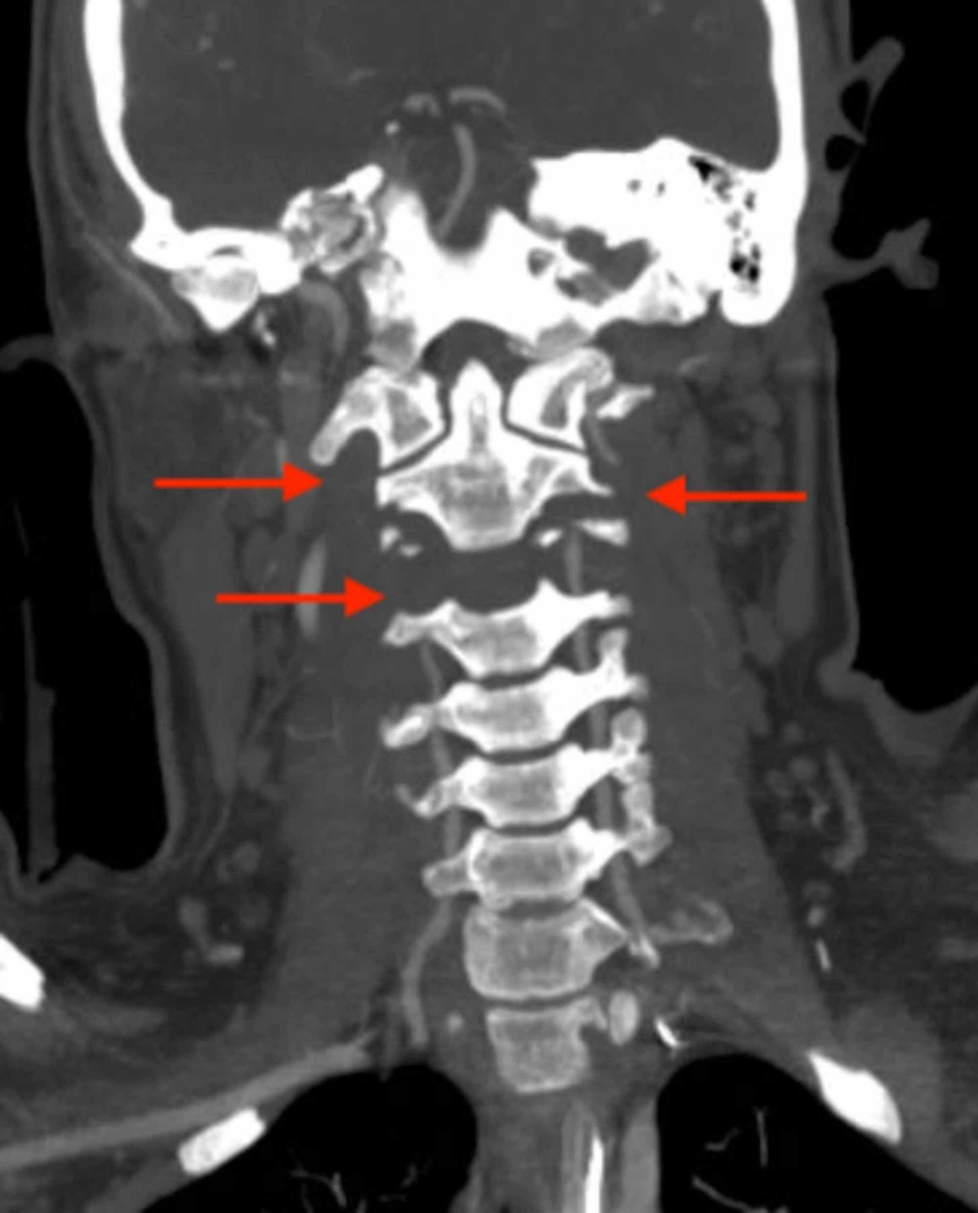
CT angiography of the cervical spine: coronal view red arrows show bilateral vertebral artery injury at the level of C2.
